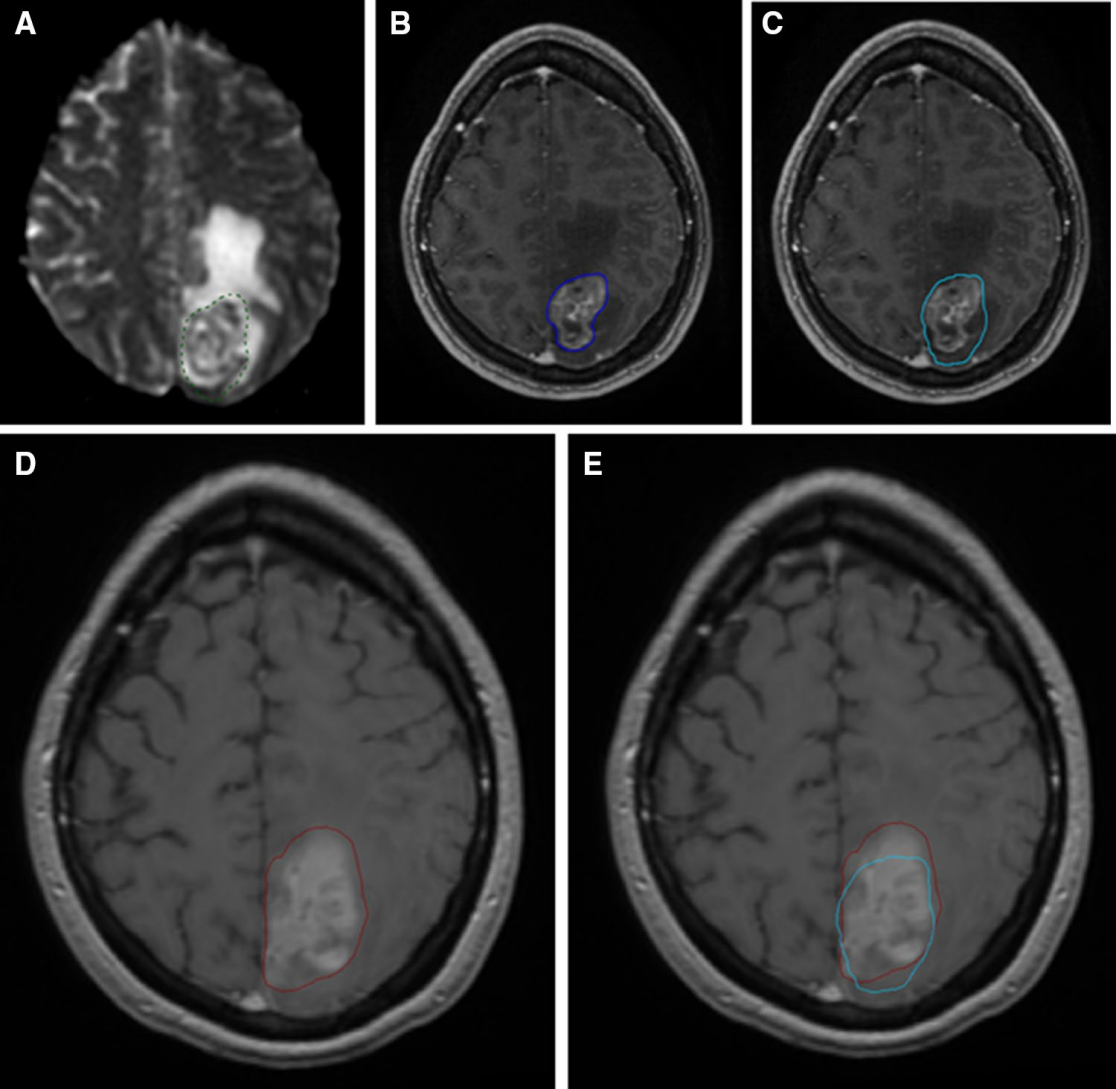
An occipital metastasis in a patient with metastatic melanoma (M-011). Treatment plans were generated based on a the ADC map—GTV_ADC_ and b the post-contrast T1-weighted planning study—GTV_T1gad_. The combined plan with addition of these two volumes is termed the diffusion treatment volume or DTV (c). These volumes are superimposed on post-contrast T1-weighted sequences acquired at the point when the resected tumour recurred. The volume of local recurrence that was covered by the GTV_T1gad_ (d) was less than that covered by the DTV (e) and differed in conformation
